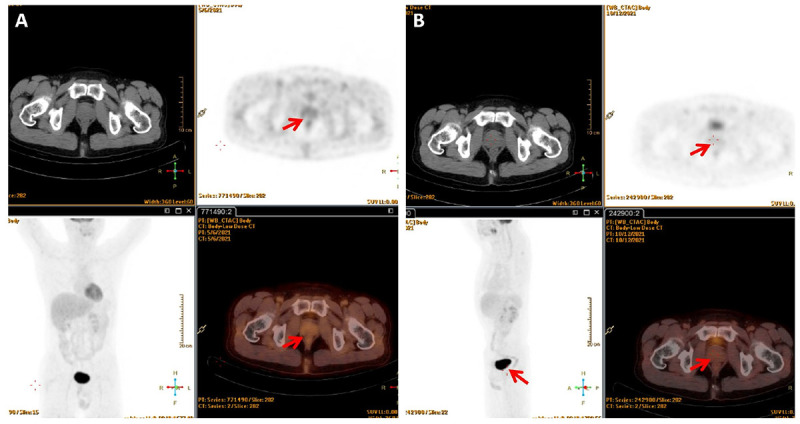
18F-FDG PET/CT showed no abnormal increased glucose metabolism in the lesion (A) 3 months after radiotherapy; (B) 6 months after radiotherapy.
